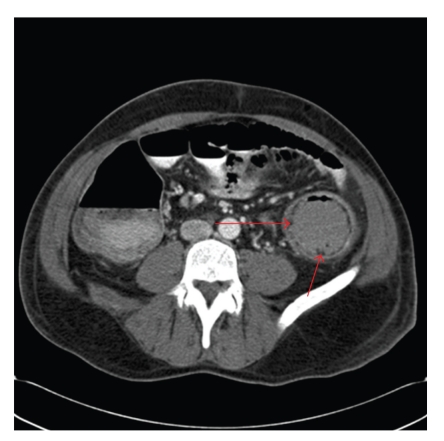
Abdominal computed tomography (CT) revealing submucosal air-fluid cysts. Arrows demarcate submucosal gas within the wall of the left colon, pneumatosis coli.
